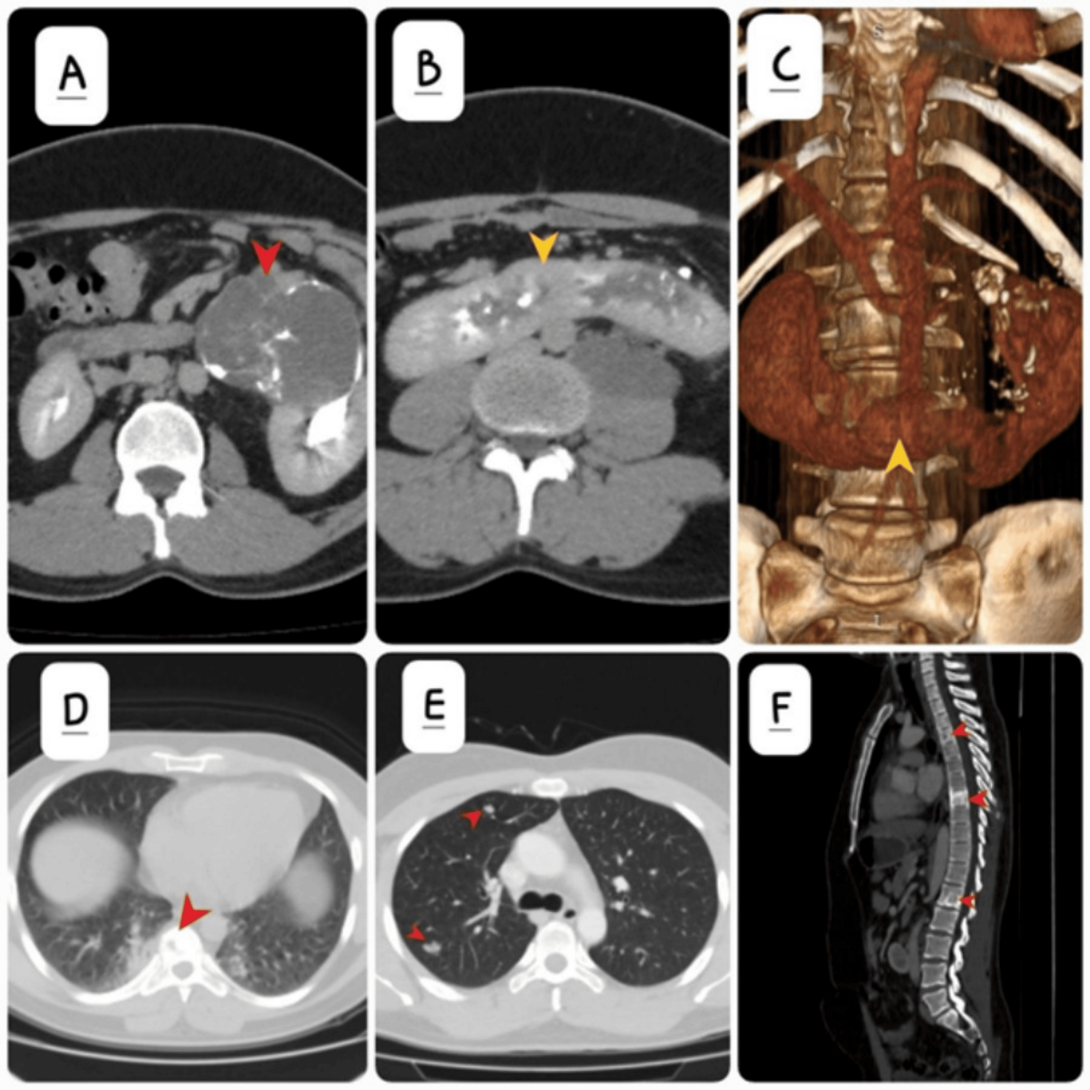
(A) Axial contrast-enhanced CT (CECT) scan showing left renal mass with multifocal coarse calcification (red arrow). (B) Axial CECT demonstrates the horseshoe kidney with yellow arrow annotating the stump. (C) 3D volume rendered coronal image again demonstrates the horseshoe kidney and dense foci of calcification in the left lumbar fossa. (D) Axial CECT image with a lytic lesion in the thoracic vertebra (red arrow). (E) Soft tissue pulmonary nodules suspicious for metastasis (red arrows). (F) Sagittal CT section, a bone window that shows osteoblastic lesions in the fourth and eighth dorsal vertebrae (D4 and D8) as well as the first lumbar vertebra (L1) (red arrows).
